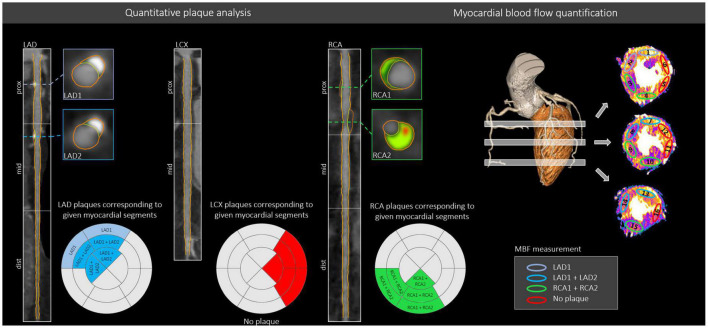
Comprehensive plaque assessment and the evaluation of myocardial ischemia based on CT images. A representative case of our study depicts the images of a 62-year-old male patient who underwent coronary CTA and DPCT imaging for the evaluation of CAD and corresponding myocardial ischemia. In this case, plaques were detected and quantified in the proximal LAD (LAD1), mid-LAD (LAD2) and proximal RCA (RCA1 + RCA2) coronary segments. All coronary plaques based on their location were matched for a given myocardial territory. MBF was quantified for all 16 myocardial segments using DPCT images. By creating a myocardial vessel territory map for each coronary segment, we could derive the total plaque volume that possibly influences the blood flow quantified as MBF on DPCT to any of the 16 analyzed LV territories (see Figure 2 for segmental classification). In this case, basal anterior (1) and basal anteroseptal (2) myocardial segments were influenced by the proximal LAD (LAD1) lesion, while the mid anterior (7), mid anteroseptal (8), apical anterior (13), and apical septal (14) myocardial segments were affected by two lesions: LAD1 and LAD2 (mid LAD segment) as they are located more distally. No plaques were present in the LCX. Regarding the RCA, two plaques were detected in the proximal RCA segment (RCA1 and RCA2), therefore all myocardial segments corresponding to the RCA were influenced by two plaques (RCA1 and RCA2). Plaque volumes of the lesions aligned for a given myocardial segment were summed and accounted for in the analysis, while the highest degree of lumen area stenosis of the corresponding lesions was included in the analysis. Light blue color indicates LV segments related to proximal LAD lesion, dark blue color indicates LV territories related to both proximal and mid-LAD lesions, whereas green color shows myocardial territories corresponding to the RCA lesions. LCX demonstrated no coronary lesions. ROI-s were placed in each myocardial segment on short-axial images. CAD, Coronary artery disease; CTA, CT angiography; DPCT, Dynamic perfusion CT; LAD, Left anterior descending; LCX, Left circumflex; LV, Left ventricle; MBF, Myocardial blood flow; RCA, Right coronary artery.
